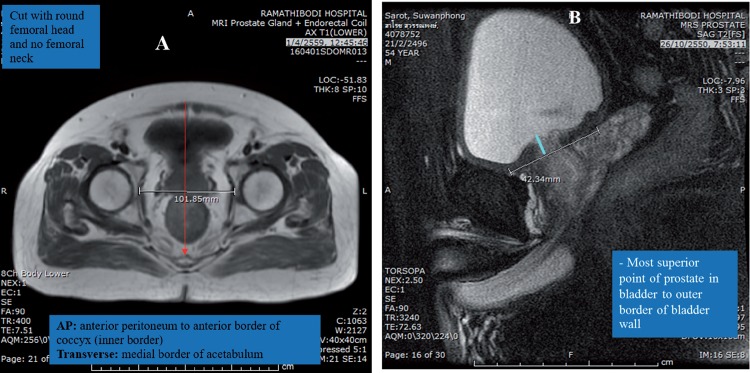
Anthropometric measurements by magnetic resonance imaging. (A) Work space transverse width (mm) on T1W image [AP: anterior peritoneum to anterior border of coccyx (inner border)] and Transverse: medial border of acetabulum; (B) Protrusion to bladder base (mm): most superior point of prostate in bladder to outer border of bladder wall.
